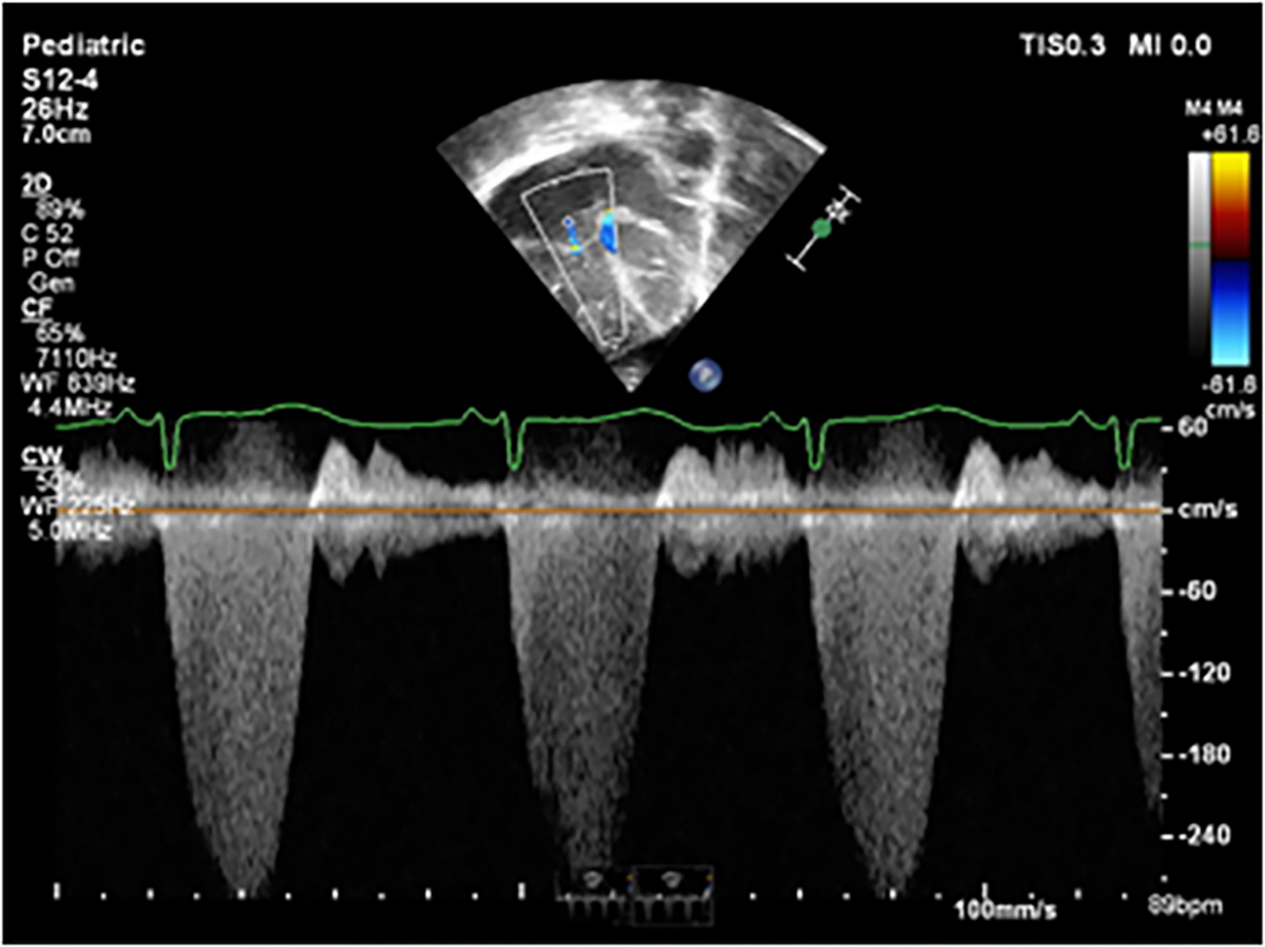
Echocardiogram continuous wave Doppler demonstrating severe tricuspid regurgitation 3.5 m/s.
